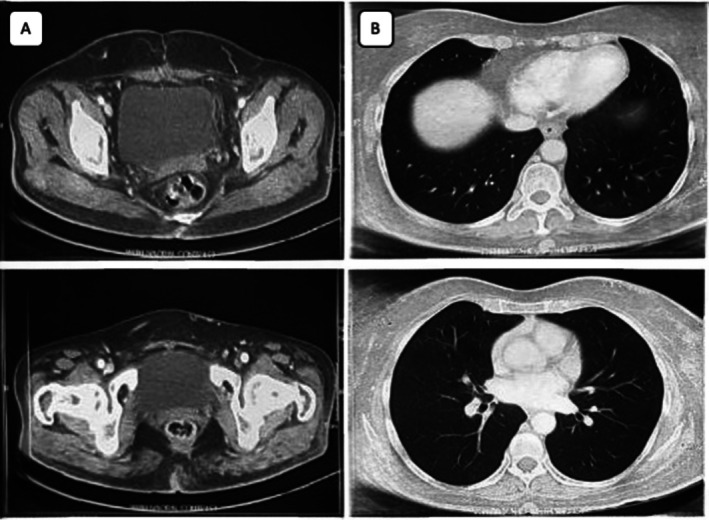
CT of the chest, abdomen, and pelvis obtained after the completion of the fourth cycles of chemotherapy revealed eradication of the lung and mediastinal lymphadenopathy, along with a significant decrease in the size of the pelvic and ovarian lesions.
